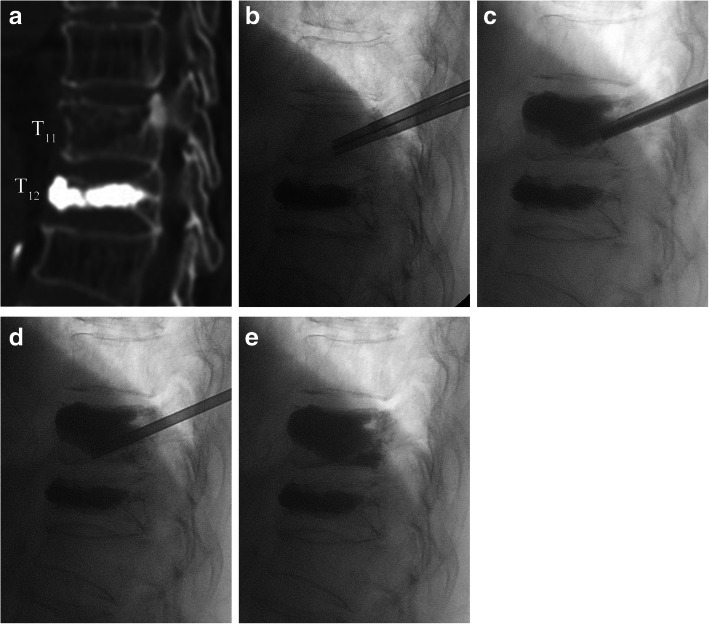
A 77-year-old female diagnosed with OVCF and a cleft underwent vertebroplasty using both a planned and a second puncture. a The preoperative sagittal CT reconstruction reveals a T_11_, large, irregular intravertebral cleft with little surrounding the cancellous bone, and the fact that T_12_ had undergone vertebroplasty 1 year prior. b The trajectory of the initial needle puncture lay along the junction between the cleft and peripheral cancellous bone and thus could be easily adjusted if a second puncture was necessary. c After initial cement injection, the cement was restricted to the cleft. d Redirecting the needle to the region lacking cement. e Final cement injection featuring intra- and extra-cleft dispersion
